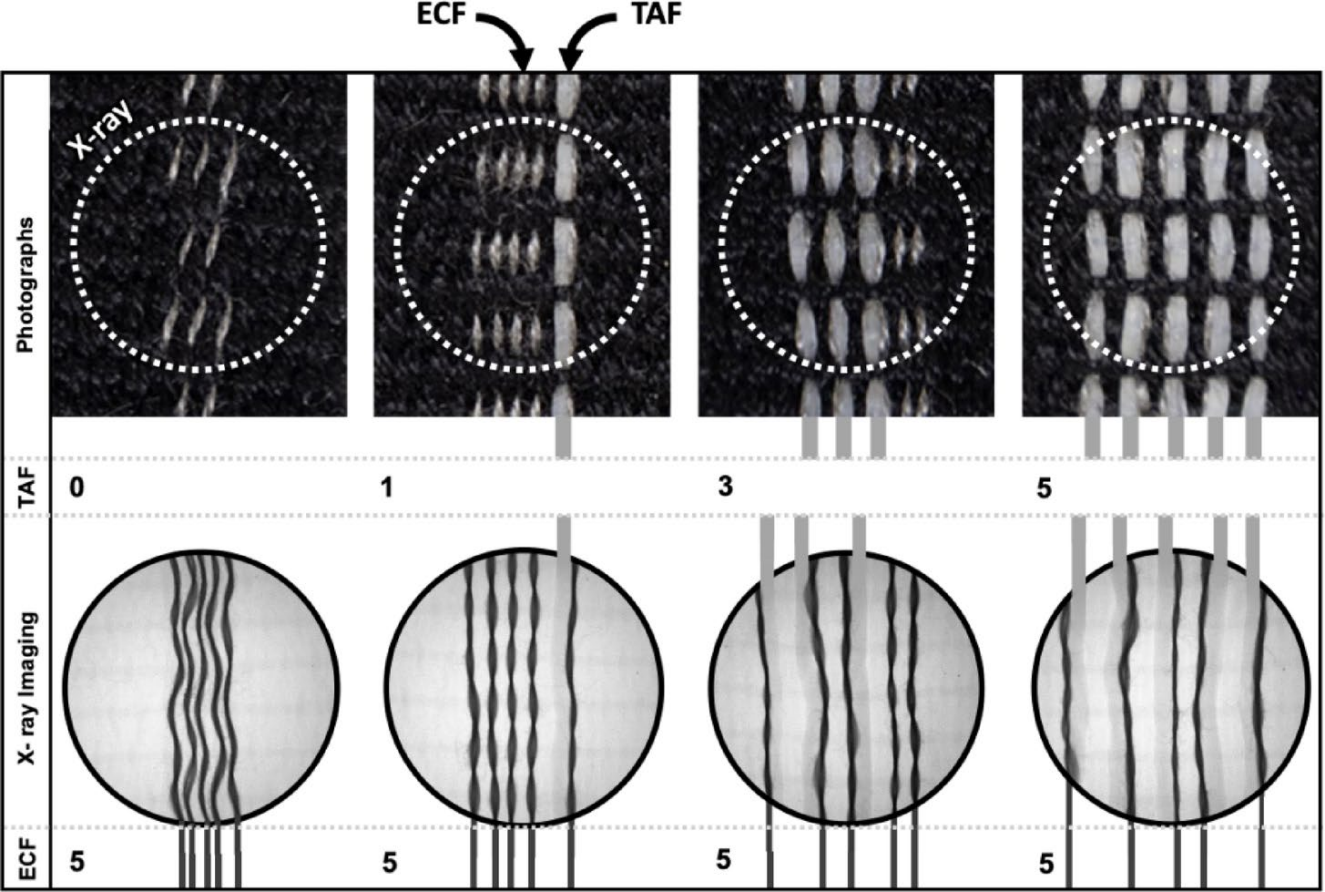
Photographs and their corresponding X-ray transmission images of four samples with zero, one, three, and five thermally actuating fibers (TAF), and five electrically conductive fibers (ECF). In these images, the circular regions each measure ca. 10 mm in diameter.
